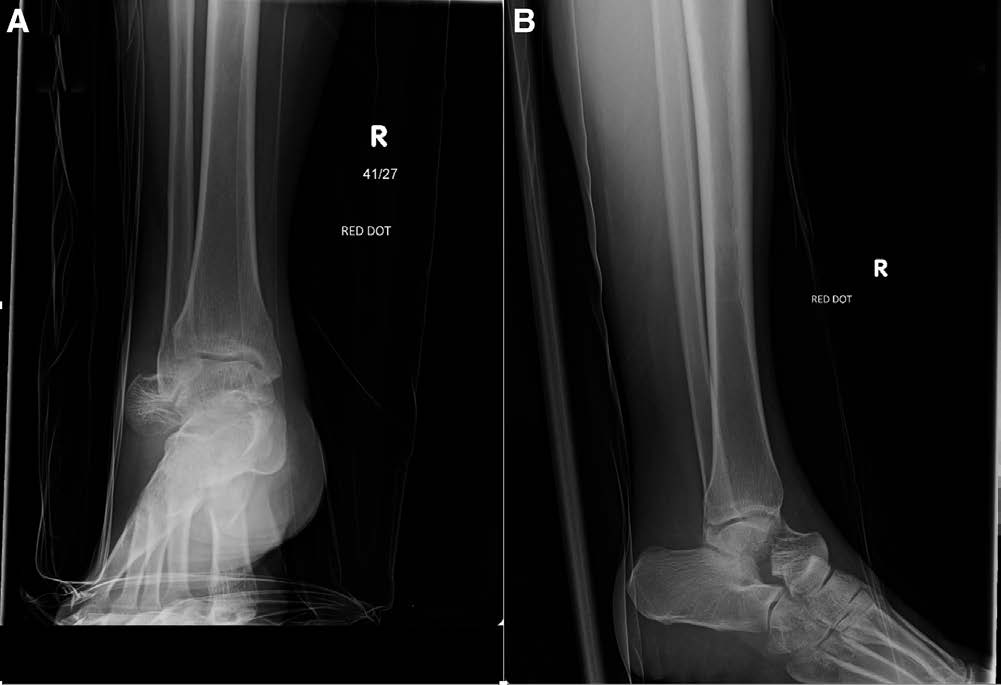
Ankle X-rays at the emergency department. (A) Anteroposterior (AP) and (B) Lateral (LAT) views showing the injury.
